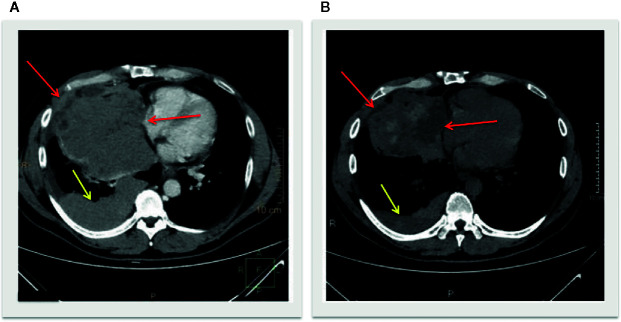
Longitudinal slices of computed tomography scans at baseline (A) and post treatment (B) showed a decrease of the mediastinum mass with intralesional hypondensity (red arrows) and pleural nodules (yellow arrows), defined as partial response according to iRECIST criteria.
